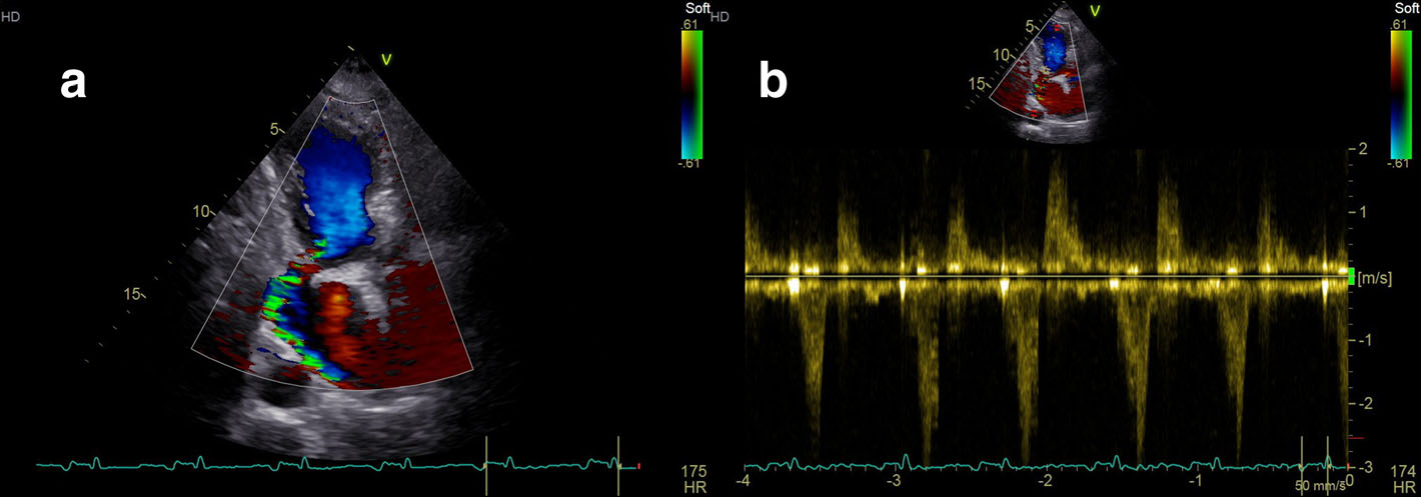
a Color Doppler imaging of transthoracic apical 3-chamber view displays the neck of the pseudoaneurysm near the mitral annulus. Systole frame shows blood flow from left ventricular into the pseudoaneurysm (blue flow). b Continuous wave doppler shows the systolic filling of pseudoaneurysm and the diastolic emptying into the left ventricular chamber (systolic velocity of 3.0 m/s and diastolic velocity of 1.7 m/s)
